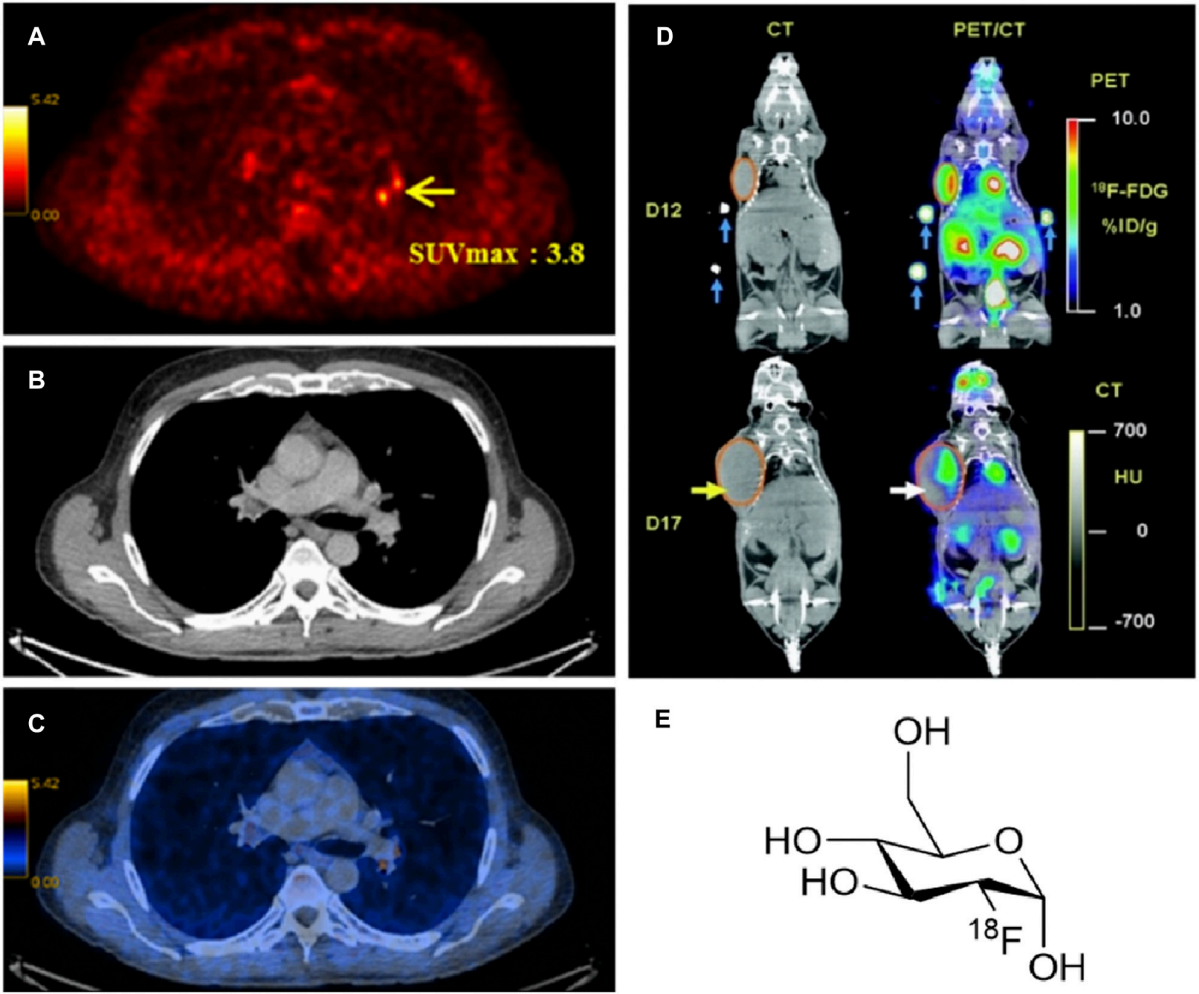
(A) PET image, (B) CT image and (C) PET/CT images of a male body with prostate cancer (Vali et al., 2015) (SUV: standardised uptake value (Kinahan and Fletcher, 2010) of ^18^F-fluorocholine). (D) Comparison between CT and PET/CT scans for the uptake of [^18^F]-FDG at day 12 and day 17. (Figure adapted from reference (Berger, 2003)), and (E) the structure of [^18^F]-FDG radiotracer.
